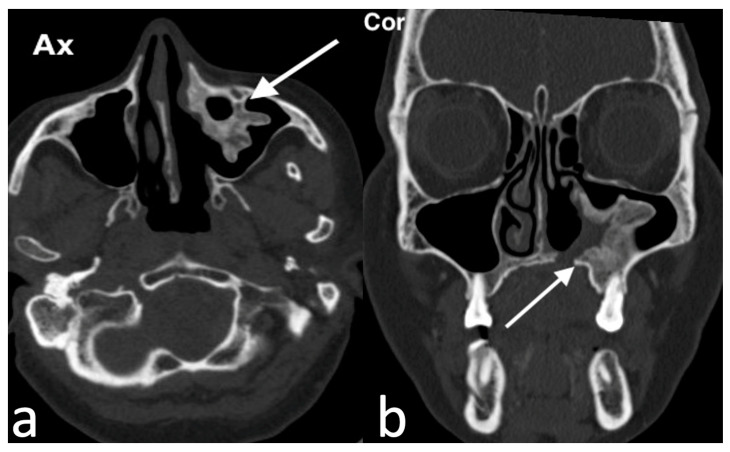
Ossifying fibroma in a 57-year-old woman with a clinical history of chronic sinusitis, who previously had undergone surgery of the left maxillary sinus. She was evaluated for the onset of painful and widespread left hemi-facial swelling, associated with fever, release of fetid purulent exudates and cacosmia. Rhinoscopy showed a lesion of bone consistency covered with normal-appearing mucosa occupying the lower portion of the left nasal cavity. Axial CT image (a) shows a lobulated and well-circumscribed mass, peripherally ossified (arrow); on coronal CT image (b), the mass (arrow) involves the lateral wall of left nasal cavity and caudally extents into the maxillary alveolar process in the periapical region of molar tooth 26. The patient underwent endoscopic re-excision of the lesion.
